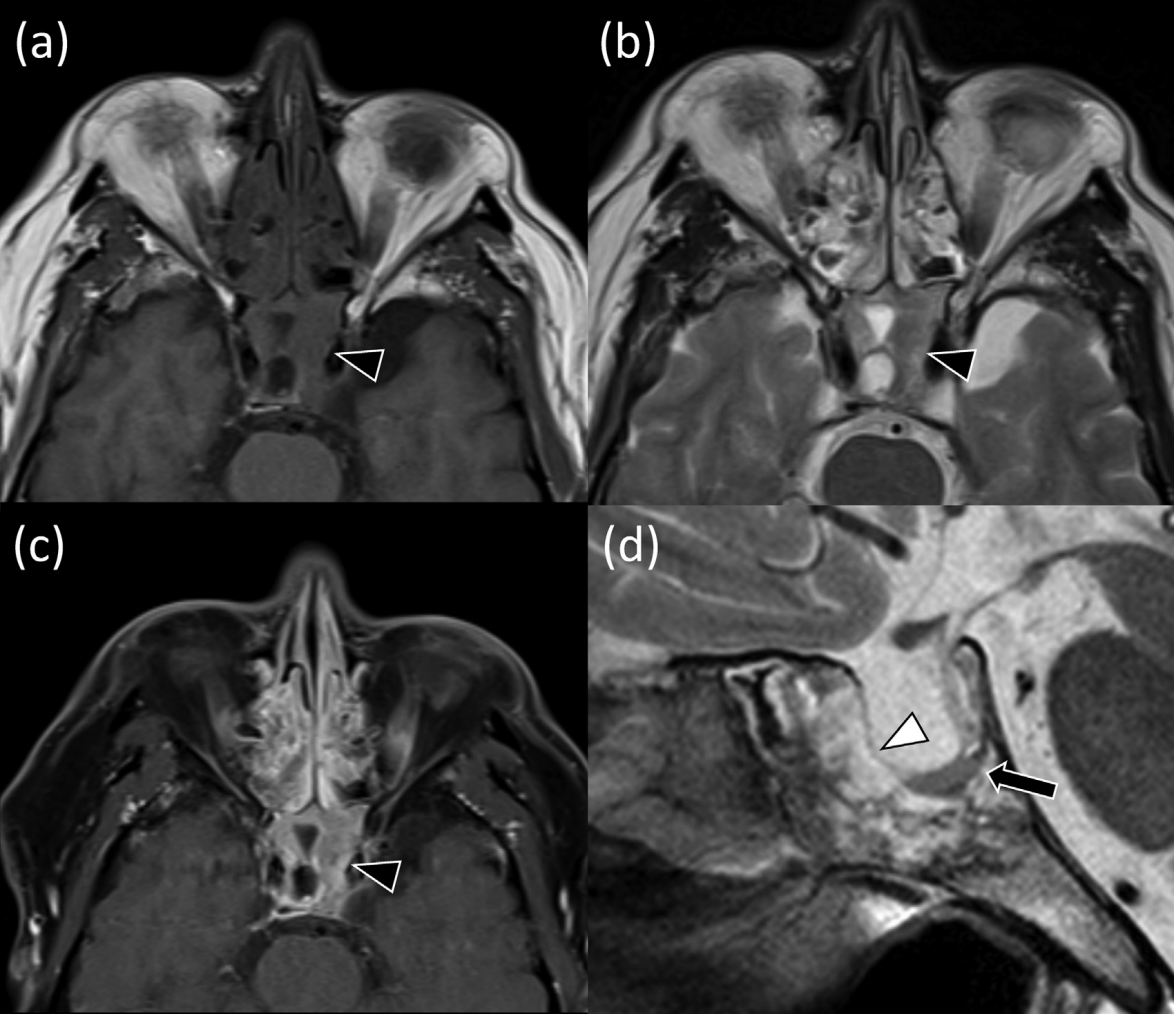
The mass in the sphenoid sinus on MRI. There is a mass in the sphenoid sinus with heterogeneous and intermediate signal intensity on T1-and T2-weighted images extending in the bilateral cavernous sinus (A, B, black arrowheads). Postcontrast T1-weighted image shows heterogeneous enhancement in the solid components and cystic components (C, black arrowhead). Sagittal T2-weighted image shows a thin anterior pituitary lobe in the basal portion of the enlarged sella turcica (D, arrow). T1-weighted hyperintensity area corresponding to the normal posterior pituitary lobe is absent (not shown). A thin T2-weighted hypointensity linear structure indicating a sella turcica dura mater is identified (D, white arrowhead).
